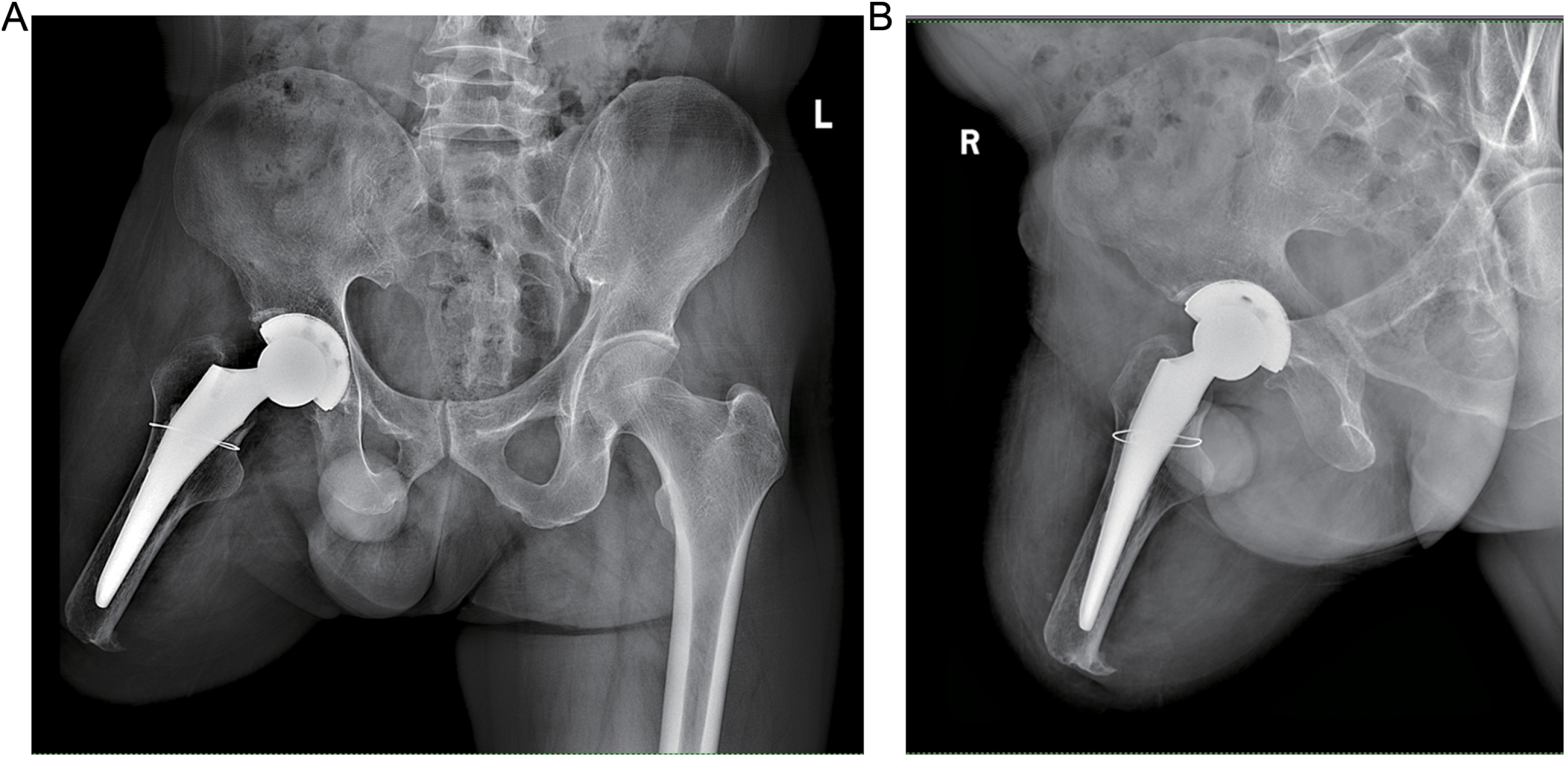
Postoperative radiograph of the patient at 5 months. (A) Anteroposterior pelvic x-ray. (B) Anteroposterior x-ray of the right hip joint.
